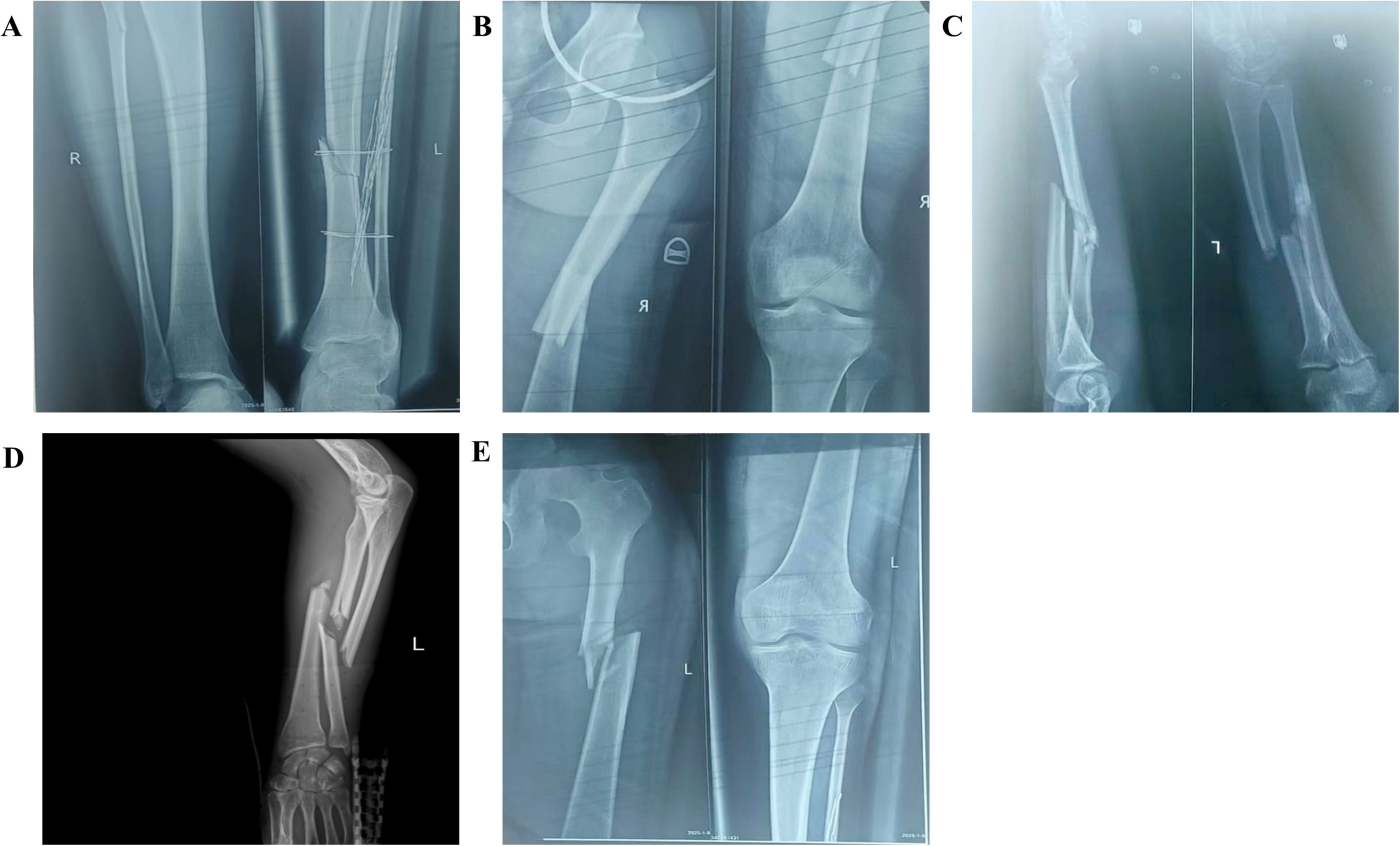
Preoperative radiographic findings: (A) Fracture of the right fibula (proximal, non-displaced); fracture of the left tibia (distal third, displaced); (B) Fracture of the right femur (midshaft, significantly displaced); (C,D) Left radial and ulnar fractures (midshaft, both displaced); (E) Fracture of the left femur (proximal third, displaced).
